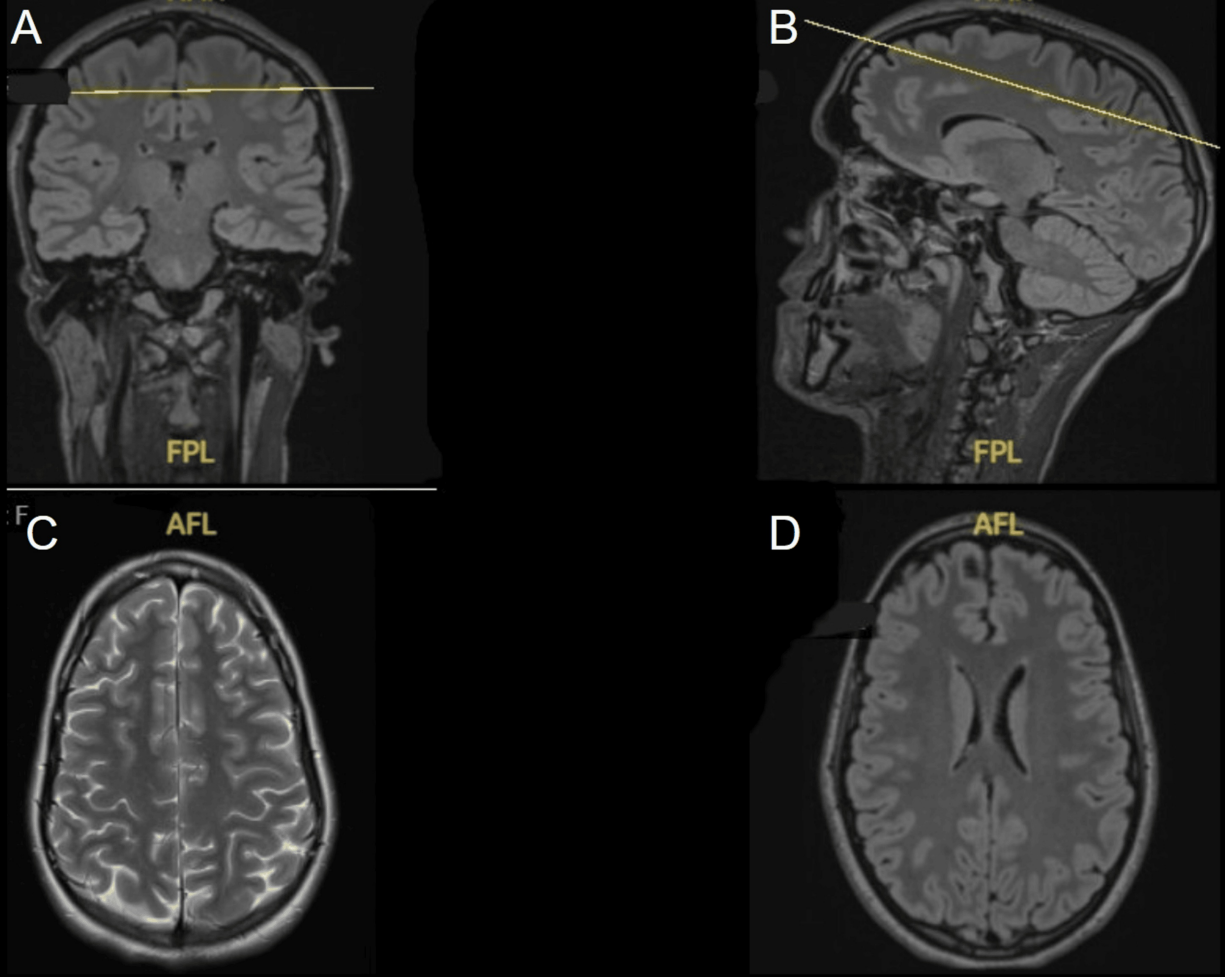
MRI of the brain (A–D) demonstrating normal sagittal, coronal, and axial views. No evidence of focal lesion. No findings to suggest inflammatory/demyelinating pathology.
